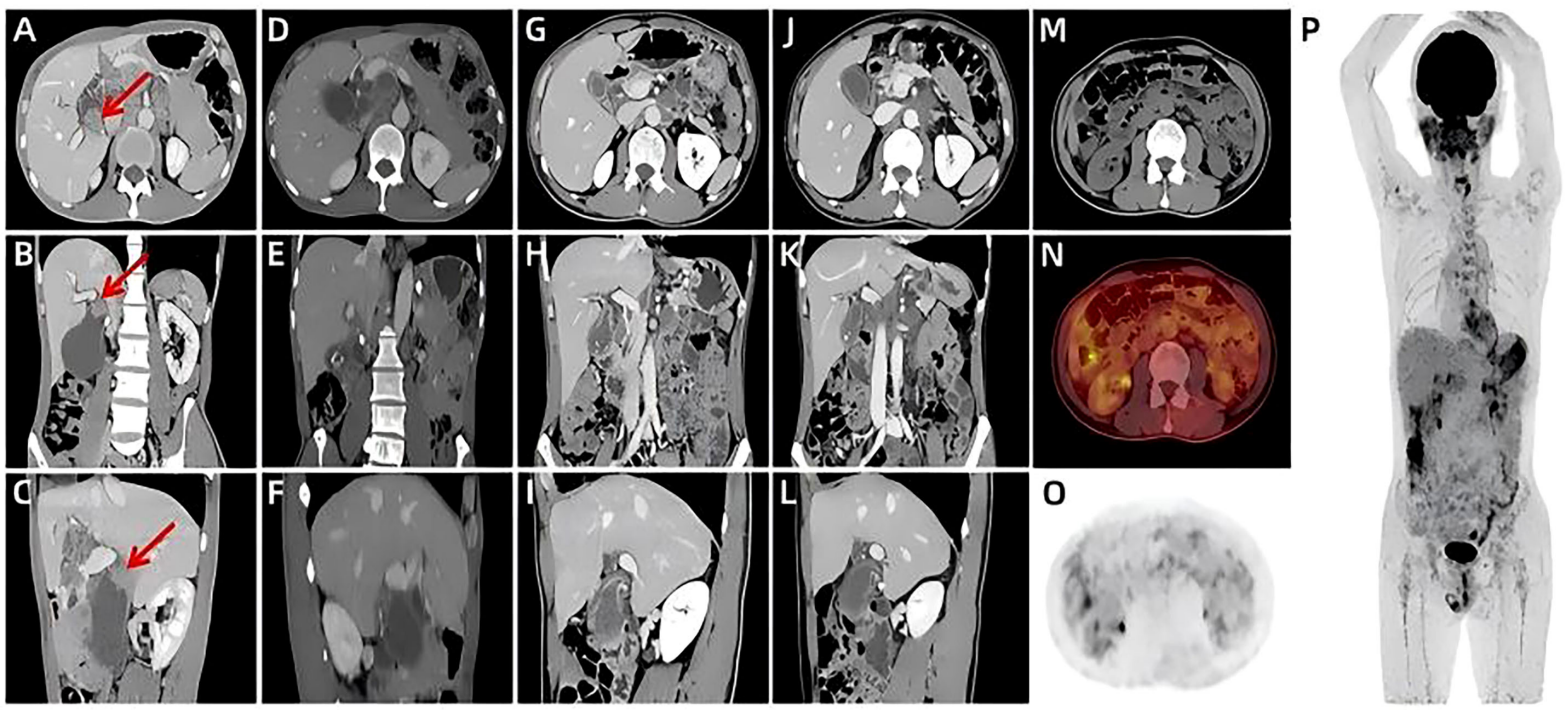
Abdomen computed tomography images: (A–C) Baseline before treatment (red arrows),contrast-enhanced CT scans at admission revealed a mass in the gallbladder neck and enlarged lymph nodes; after treatment, follow-up CT scans at one (D–F), three (G–I) and six months (J–L) post-admission, along with a PET-CT at seven months (M–P), demonstrated complete resolution of the primary lesion and reduction of lymphadenopathy.
